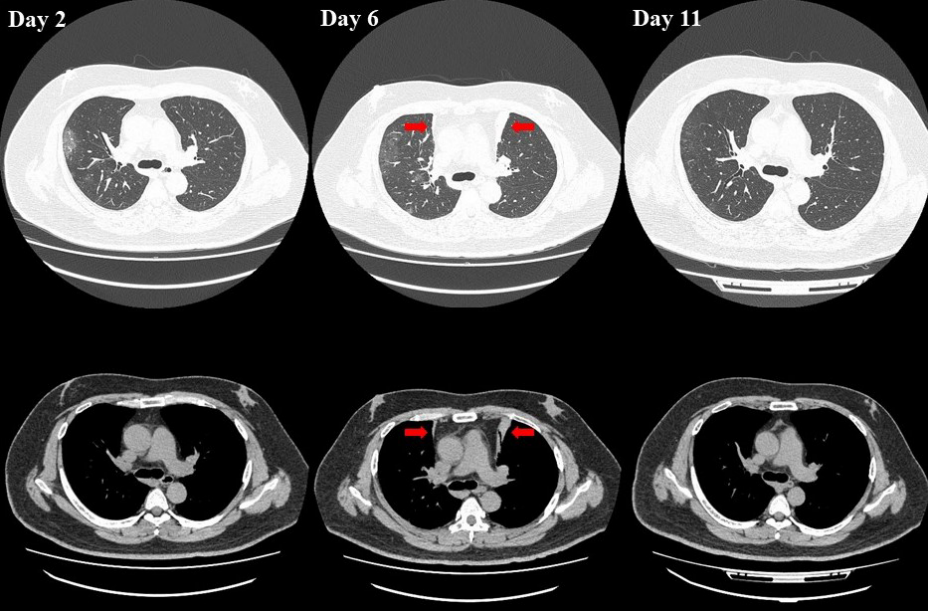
Results of chest computed tomography at 2, 6, and 11 days after onset.
